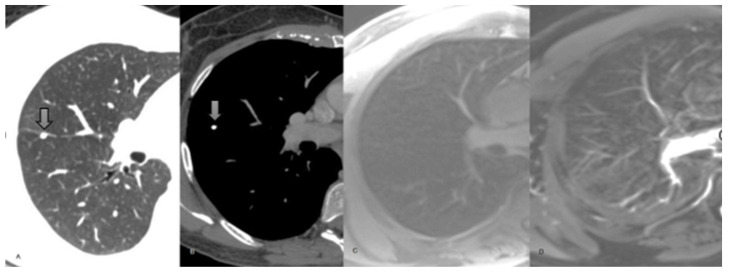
42-year-old female presenting with renal cancer. (A) Axial non-enhanced CT chest, lung window. (B) Axial T1-weighted non-enhanced chest MRI. Both images show a 5 mm calcified perifissural nodule. (C) Axial T1-weighted chest MRI. (D) Axial T2- weighted chest MRI. The calcified nodule demonstrated in the chest CT scan is missed in the MRI. The nodule was stable for 6 months and was considered benign.
